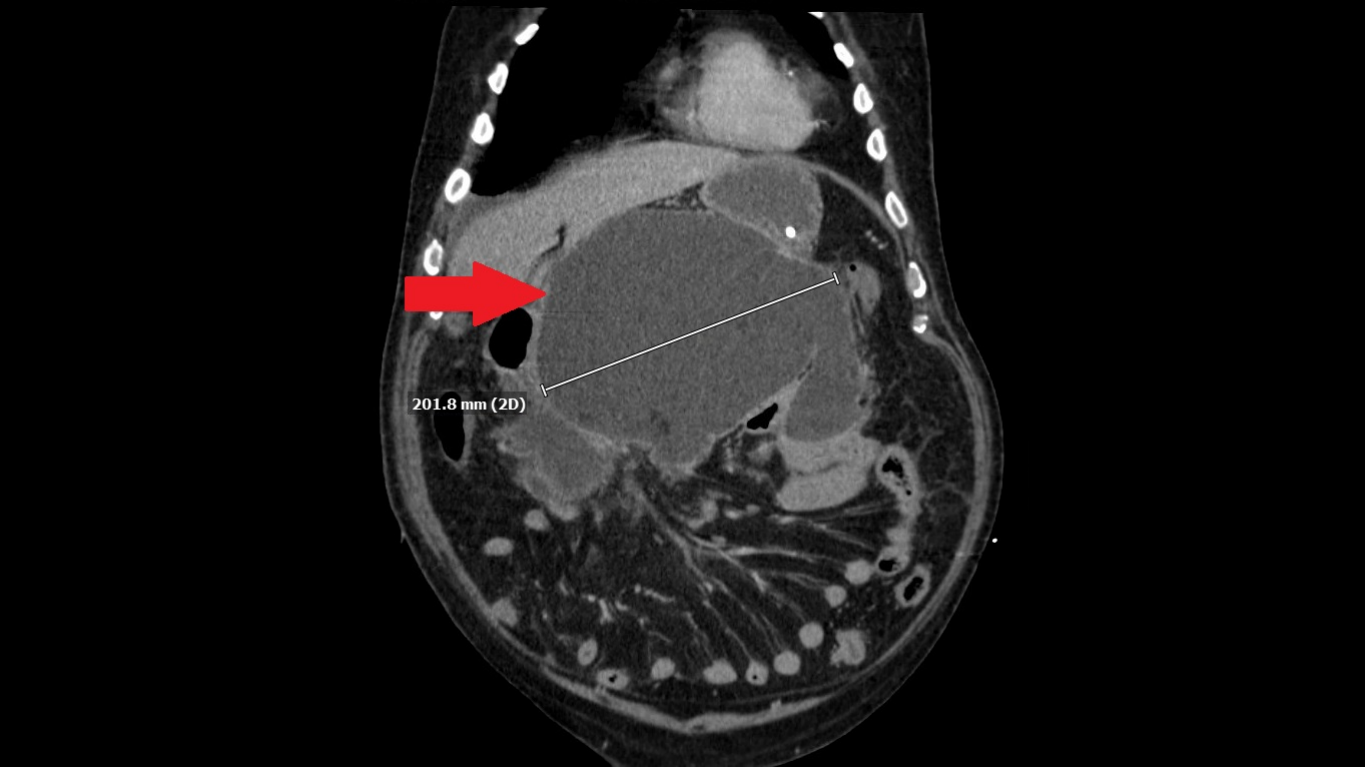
Computed tomography of the abdomen - coronal view Computed tomography of the abdomen showing a coronal view of a large pancreatic pseudocyst
